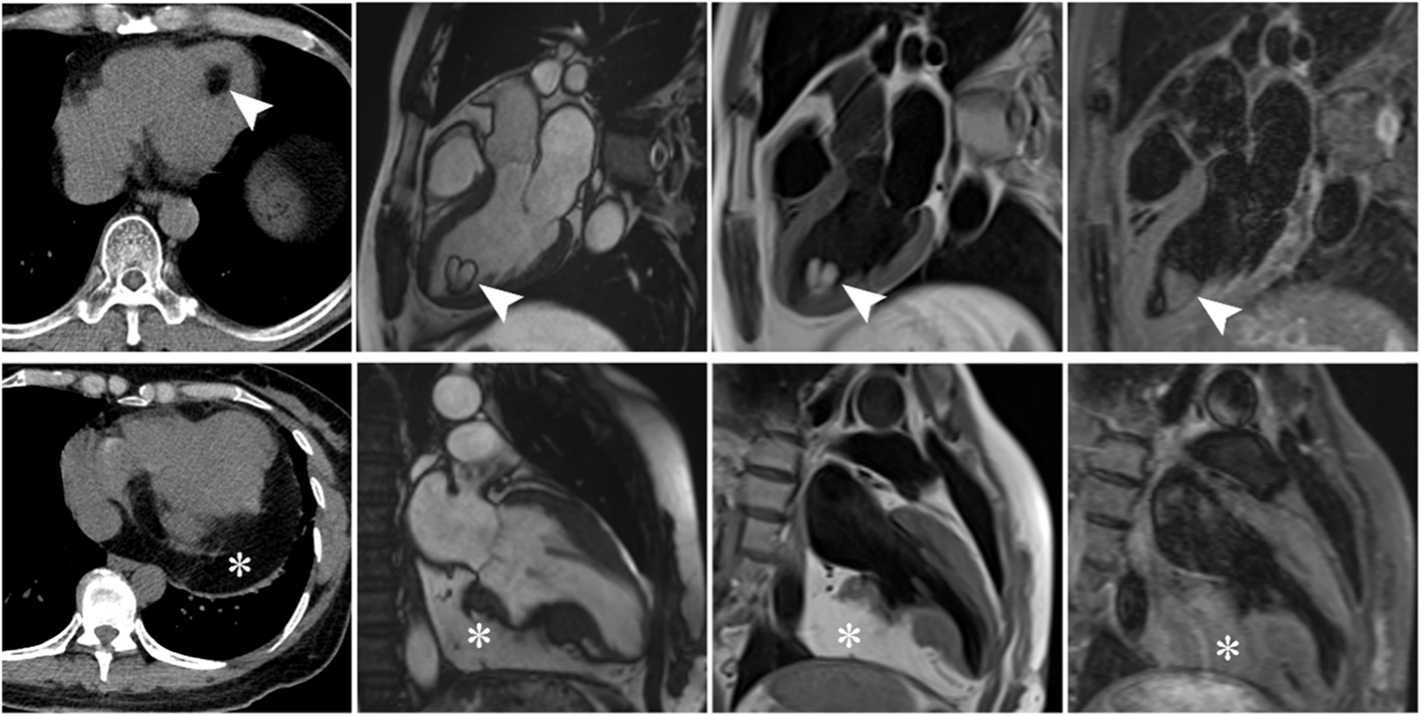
CT and MR images of left ventricular (triangular arrows) and pericardial (asterisk) lipomas. Imaging features of cardiac lipomas show consistence with subcutaneous fat in all sequences
